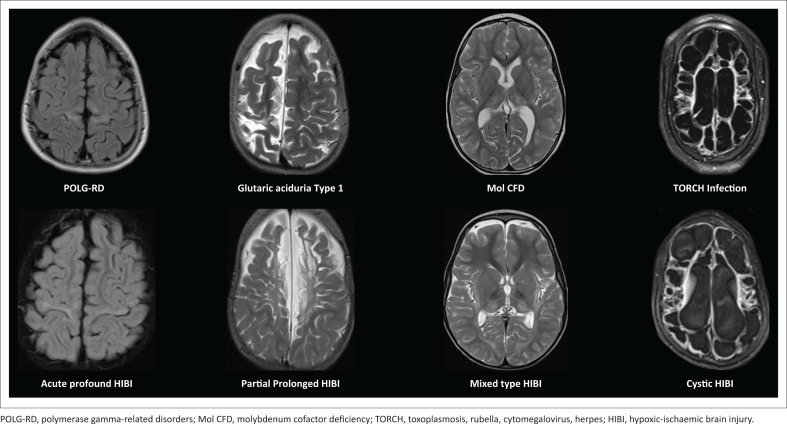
The diagnoses of the mystery cases are revealed with the bottom row listing the main subtypes of HIBI while the top row annotates mimicking conditions with often identical MRI patterns. The reporting radiologist will do well to keep this in mind when facing a challenging paediatric MRI study.
